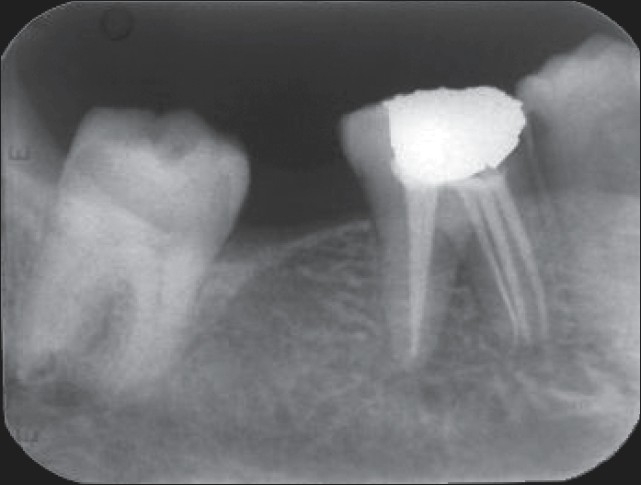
Postoperative obturation radiograph
